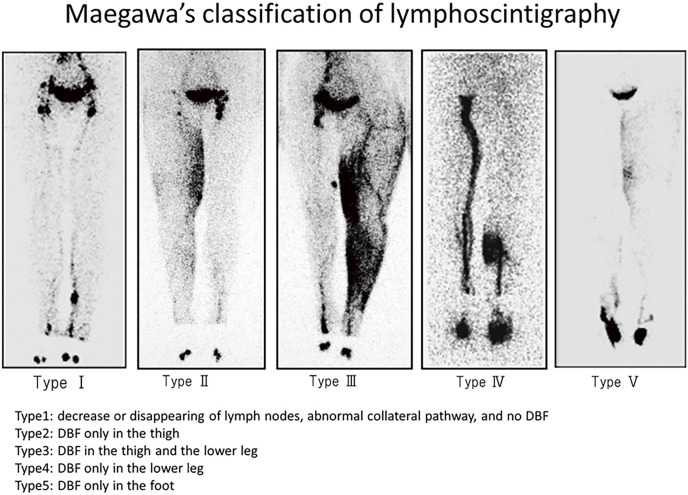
Fig. 1 Maegawa’s classification of lymphoscintigraphy. The pattern of LP and DBF changes as the lymphatic vessels degenerate progres (modified and quoted from reference 6). LP: linear pattern; DBF: dermal back flow
